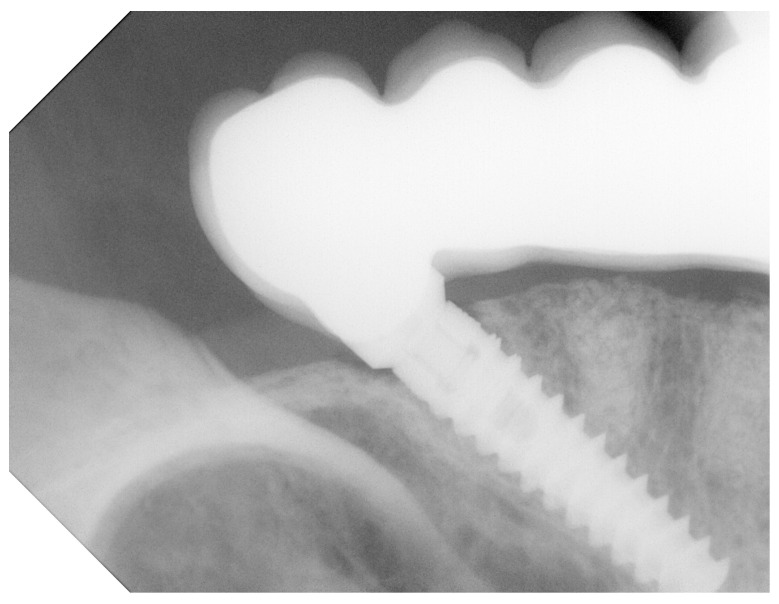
Marginal bone loss was evaluated by measuring the limbus alveolaris around the implants, by using standard right-angle parallel technique with single digital radiographs.
